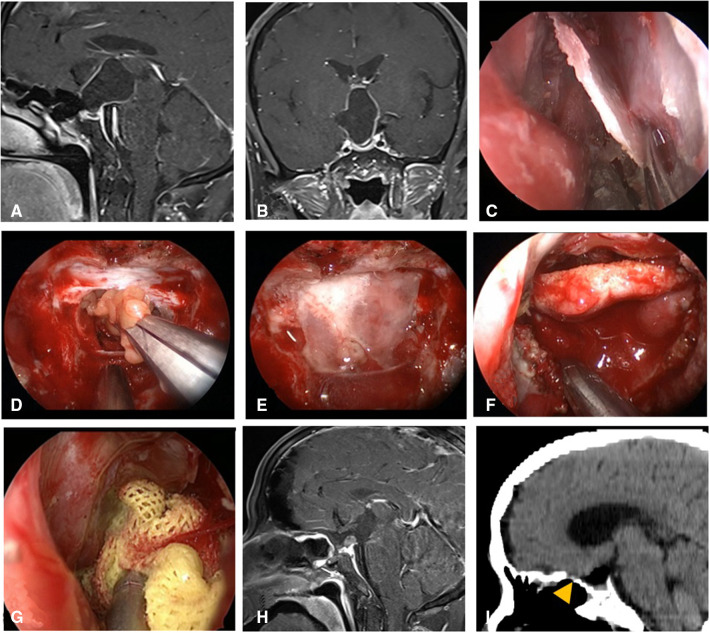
Changes in reconstruction strategy/(after) [(C) harvest of nasal septum bone flap; (D–G) process of reconstruction]. (A,B) MRI enhanced in sellar region before operation, and craniopharyngioma was considered before operation. (C) Separation of nasal septum bone flap. (D) Subdural fat packing. (E) Repair of skull base bone window with trimmed nasal septum bone flap. (F) Cover the VP-NSF on the nasal septum bone flap. (G) Gauze support. (H) MRI enhanced in sellar after operation and postoperative pathology showed craniopharyngioma. (I) CT bone window showing nasal septum bone flap was in place. VP-NSF, vascularized pedicle nasoseptal flap.
